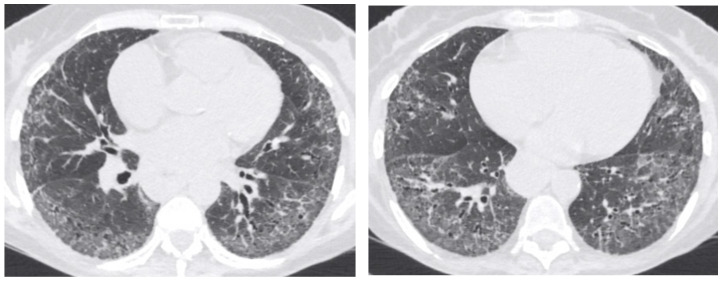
CT of the thorax—lung window—showing bilateral fine interstitial thickening and ground glass opacities with a basal predominance, minimal traction bronchiectasis and relative subpleural sparing (NSIP pattern).
